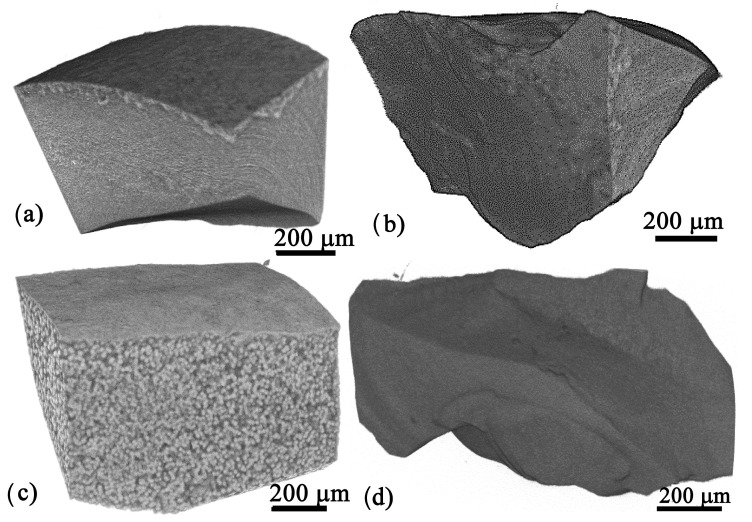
X-ray tomograms of samples with (a) 0.5 mol% and (b) 1 mol% ZrO_2_ annealed for 3 h at 590 °C; (c) 1 mol% and (d) 3 mol% ZrO_2_ heat-treated for 24 h at 590 °C.
